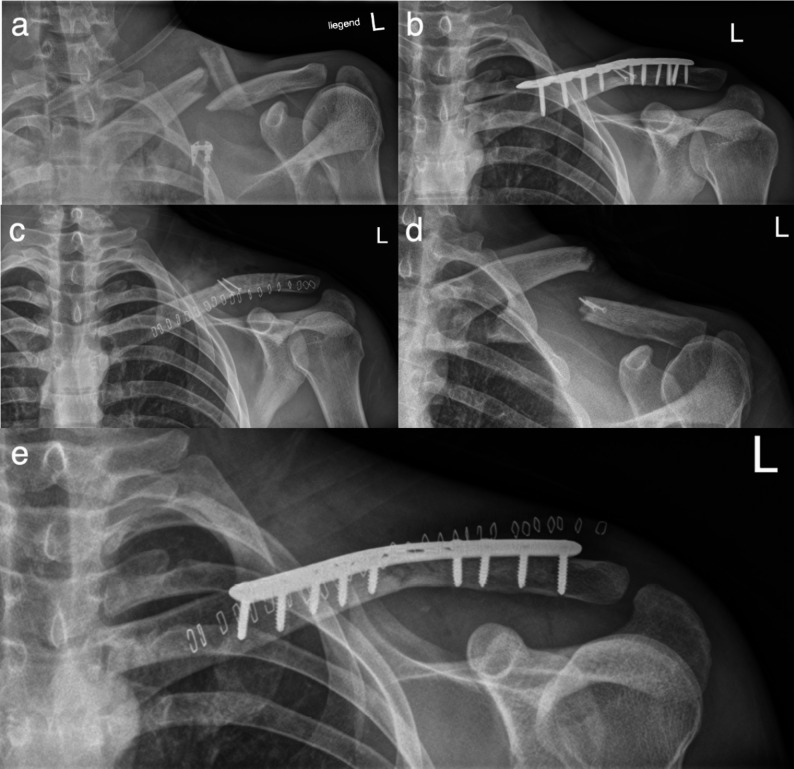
Midshaft clavicle fracture (AO 15.2C). a Preoperative radiograph (AP view); b postoperative radiograph after osteosynthesis using a locking plate with two interfragmentary lag screws (AP view); c radiograph after implant removal with the interfragmentary lag screws left in place (AP view); d refracture at the original fracture site (AP view); e postoperative radiograph after revision surgery using a locking plate (AP view)
